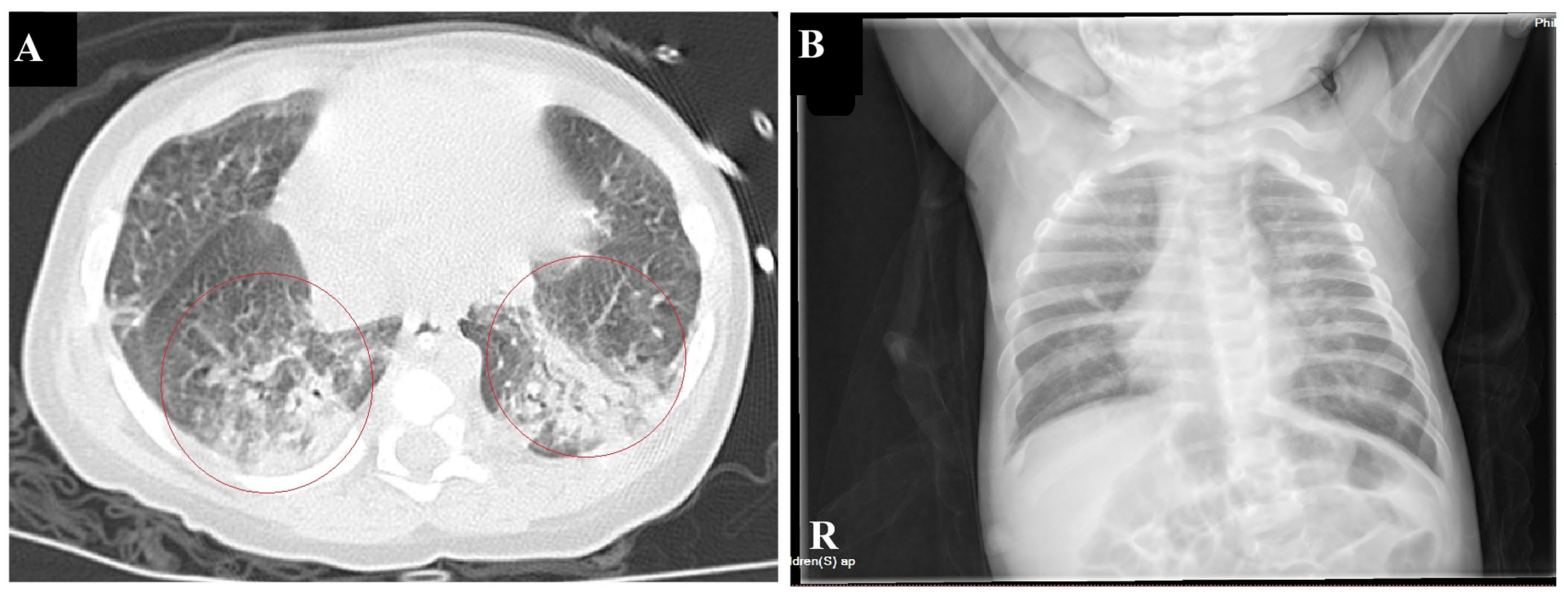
Chest imaging before discharge (A). Chest CT on January 23rd shows a significant reduction in patchy hyperdense shadows in both lungs compared to the previous imaging (B). Chest radiographs on February 9th indicate a significant improvement in lung inflammation.
